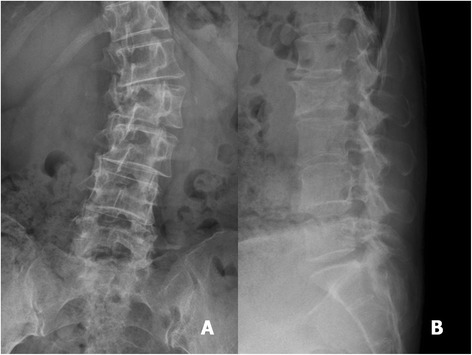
A 55-year-old woman suffered from progressive back pain with bilateral lower extremities weakness. The anteroposterior (a) and lateral (b) radiographs revealed collapse of the vertebral body of L2 with lateral segmental deviation and malalignment
